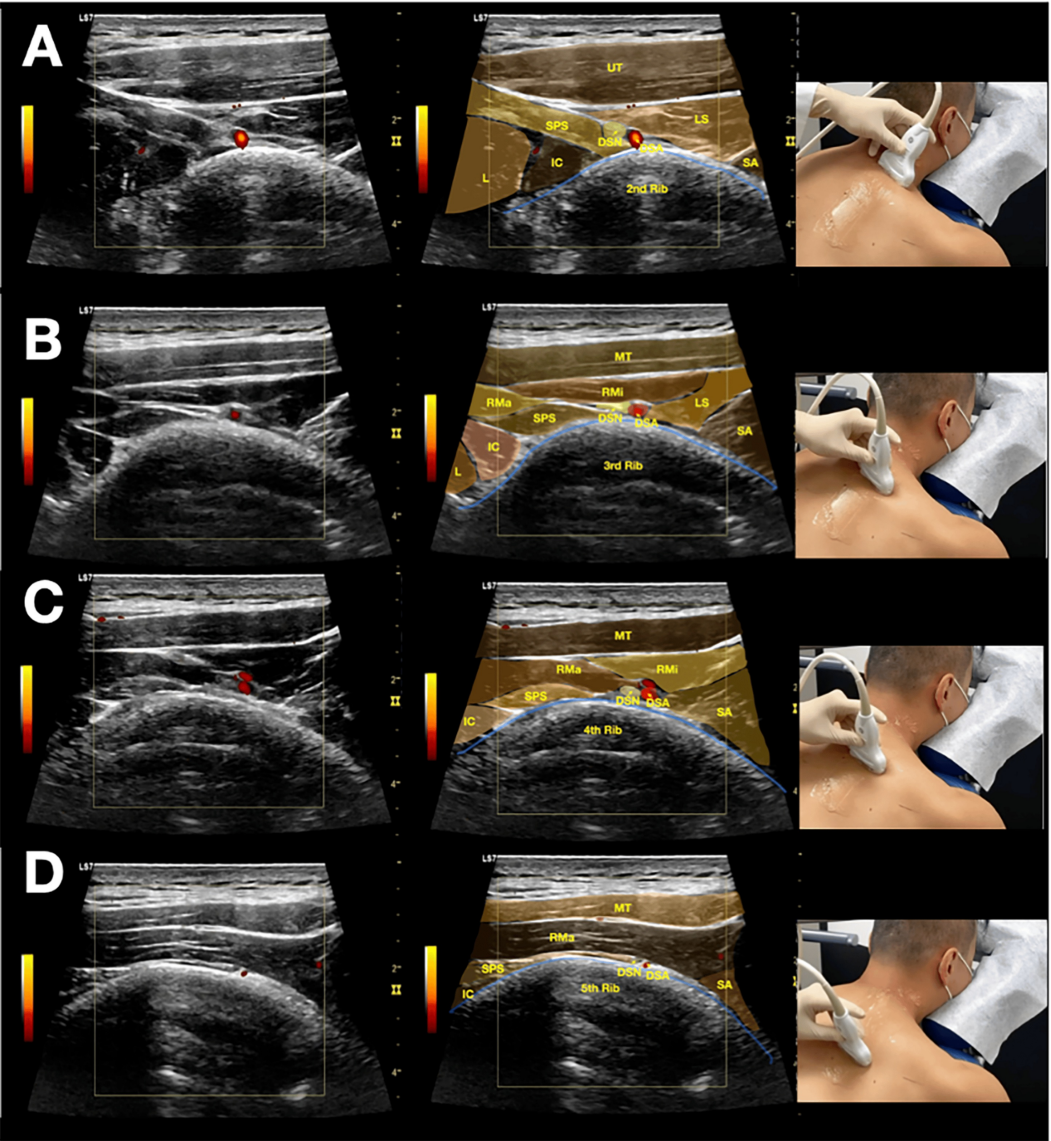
Sonoanatomy of the dorsal scapular nerve in the scapular region. Ultrasound images demonstrating the anatomical relationships for dorsal scapular nerve (DSN) identification in the interscapular region from the second to fifth rib levels. (A) DSN at the level of the second rib. (B) DSN at the level of the third rib. (C) DSN at the level of the fourth rib. (D) DSN at the level of the fifth rib. Key anatomical features include the DSN visualized as a hypoechoic oval structure located deep to the levator scapulae and rhomboid minor muscles, superficial to the serratus posterior superior muscle, and medial to the dorsal scapular artery; superficial muscles appearing as hypoechoic triangular structures; serratus posterior superior as a fan-shaped deep muscle; and ribs as hyperechoic linear structures with acoustic shadowing (see Video 3).
